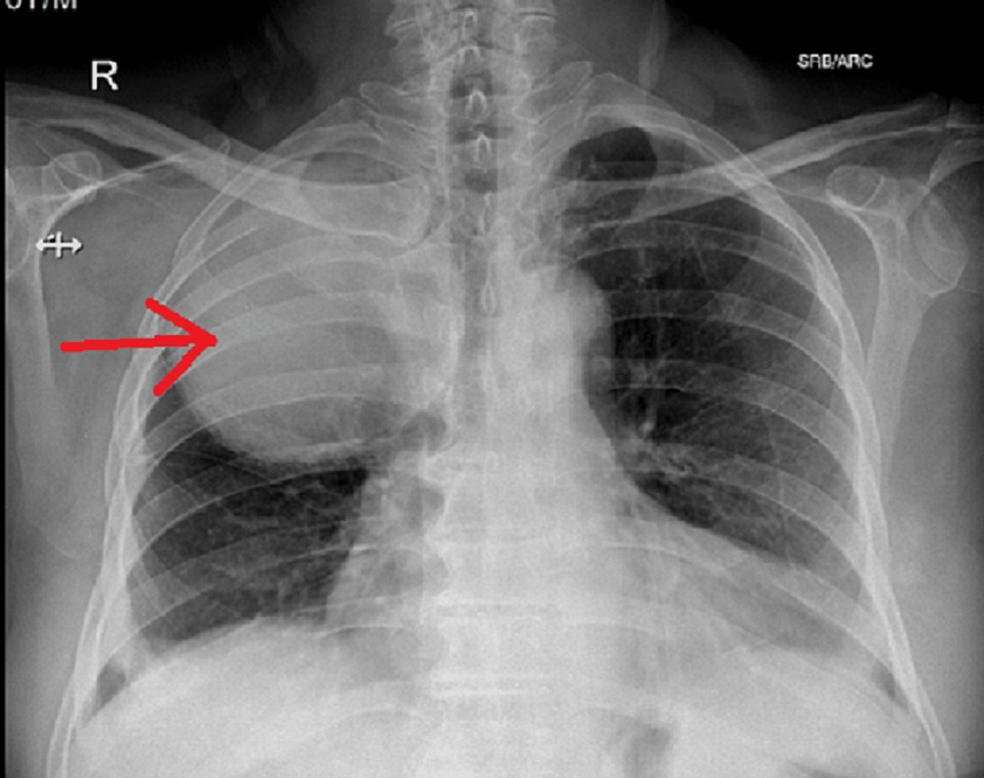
Chest X-ray (posteroanterior view) It shows a circular opacity occupying the upper and middle zone of his right lung (red arrow).
